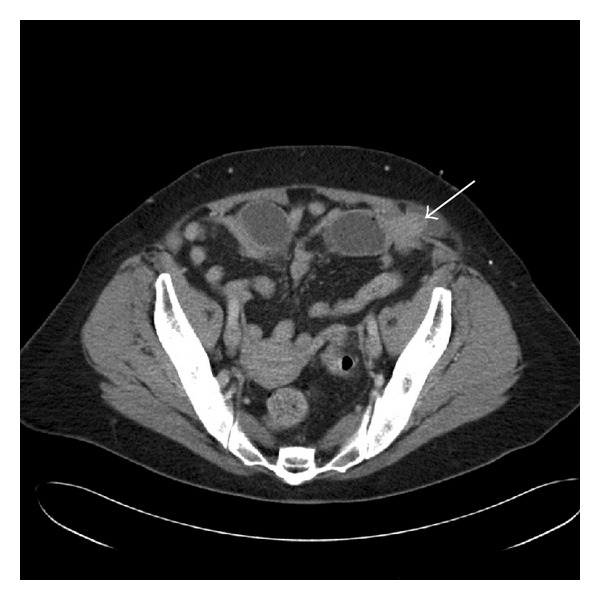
Computed tomography (CT) of the abdomen demonstrating a left-sided spigelian hernia with incarcerated loop of small bowel (white arrow points to loop of small bowel incarcerated).
